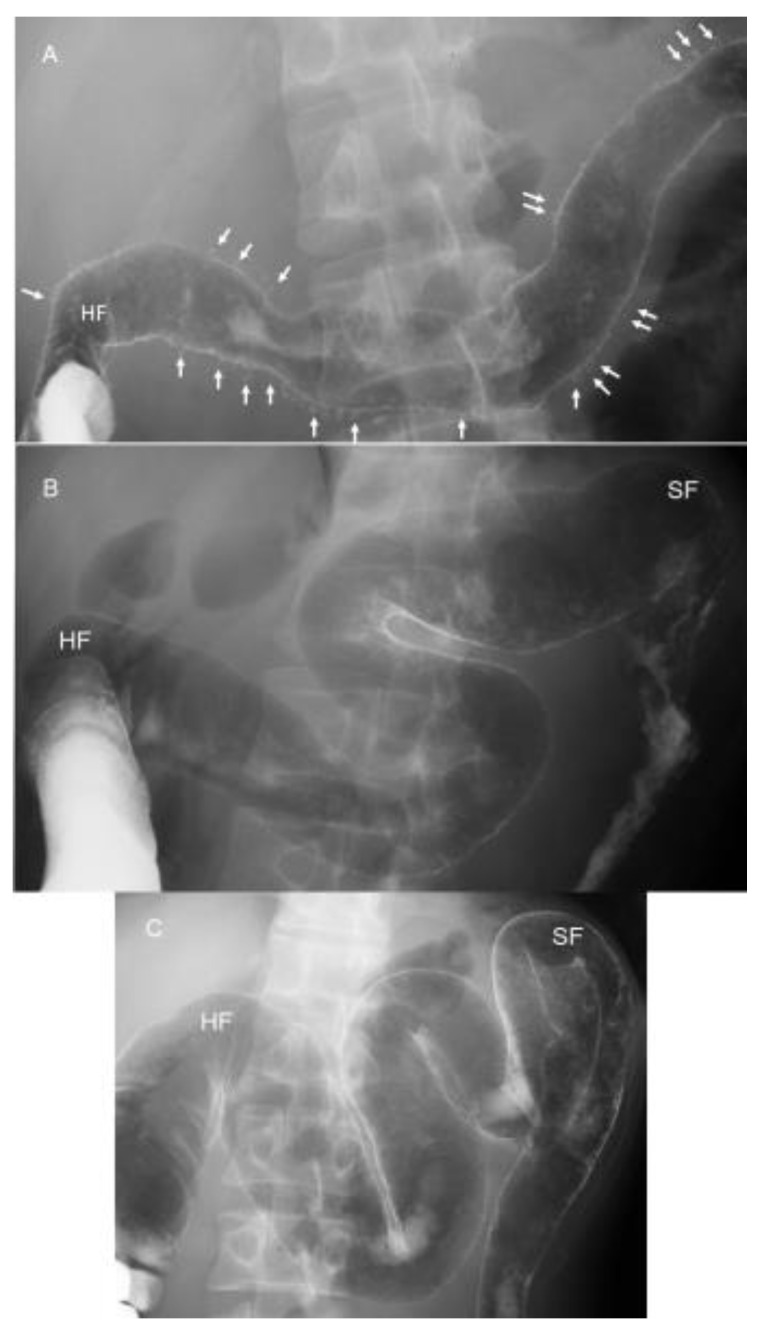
Roentgenograms of barium enema study during the induction phase in a 34-year-old man with severe ulcerative colitis (initial episode case) treated with infliximab and a plant-based diet as first-line (IPF) therapy. Numerous collar button ulcerations (arrows) are observed in the whole transverse colon 1 day before IPF therapy (A). They are resolved on the 13th day, 1 day before the second infliximab infusion (B). Further recovery with good distensibility is observed 1 day before the third infliximab infusion (C). HF, hepatic flexure; SF, splenic flexure.
